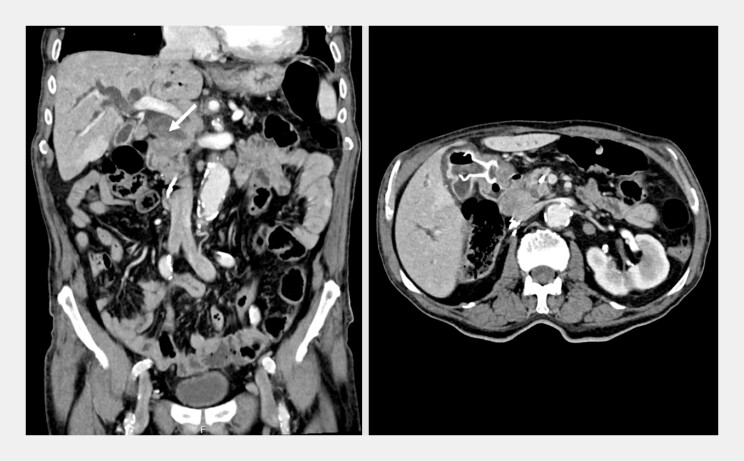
A CT scan showing biliary obstruction caused by a mass in the pancreatic head, with a previously placed cholecystogastrostomy lumen-apposing metal stent (LAMS) in situ. CT, computed tomography.
